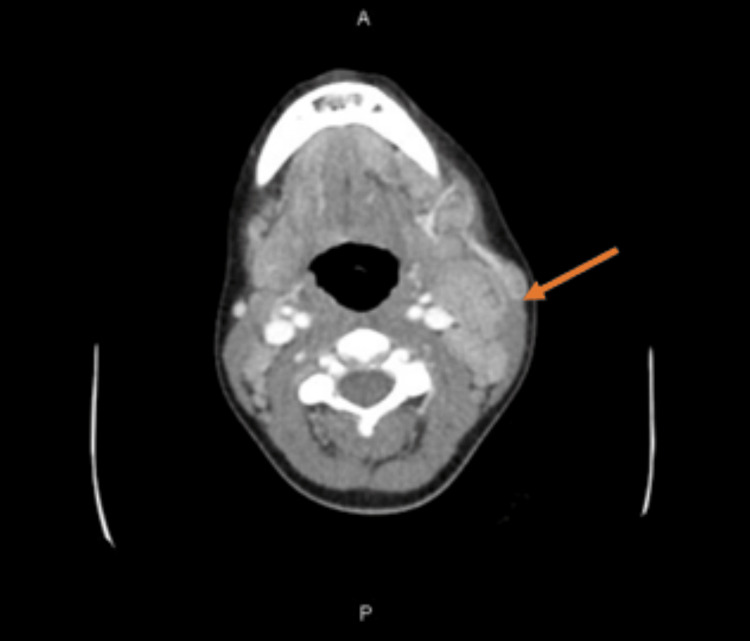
CT of the soft tissue neck with contrast The arrow points to the area of significant lymphadenopathy in the left cervical chain.
